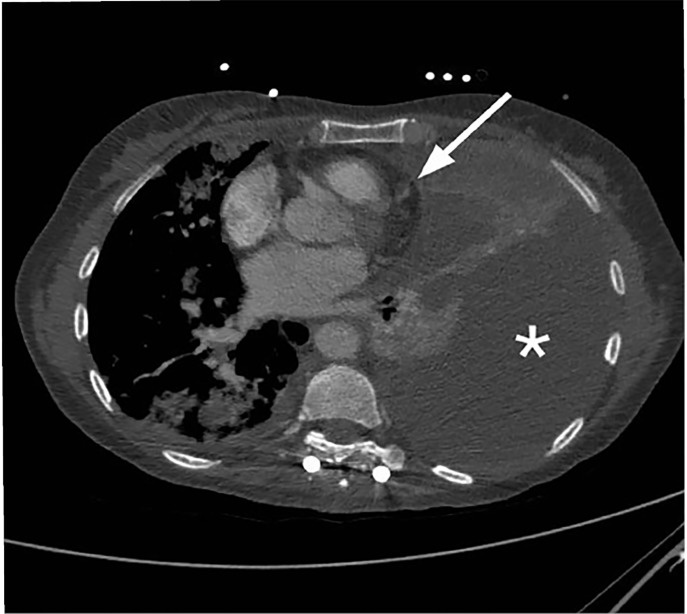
Thoracic computed tomography angiography axial view with large left pleural effusion (*) and rightward displacement of the heart (white arrow).
